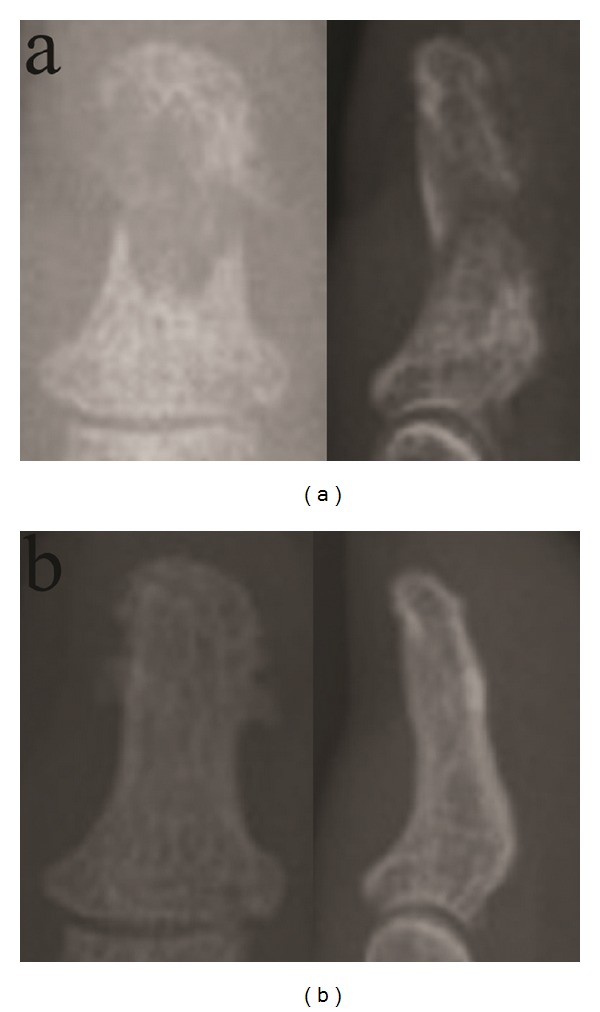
Anteroposterior and lateral radiographs at 1 month (a) and 10 months (b) after surgery.
